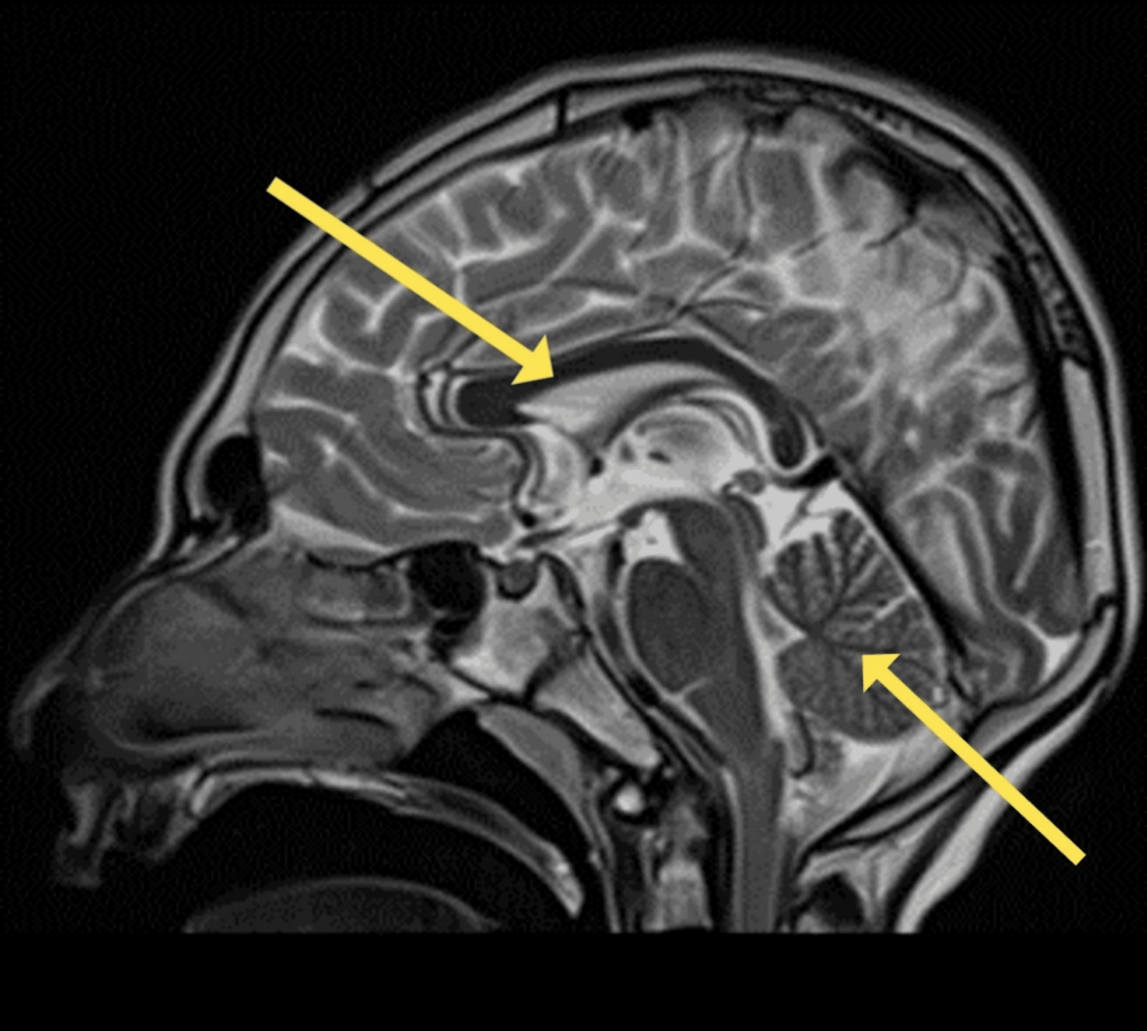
Sagittal T2-weighted magnetic resonance image revealing microcephaly and diffuse atrophy of the corpus callosum and cerebellum (arrows)
